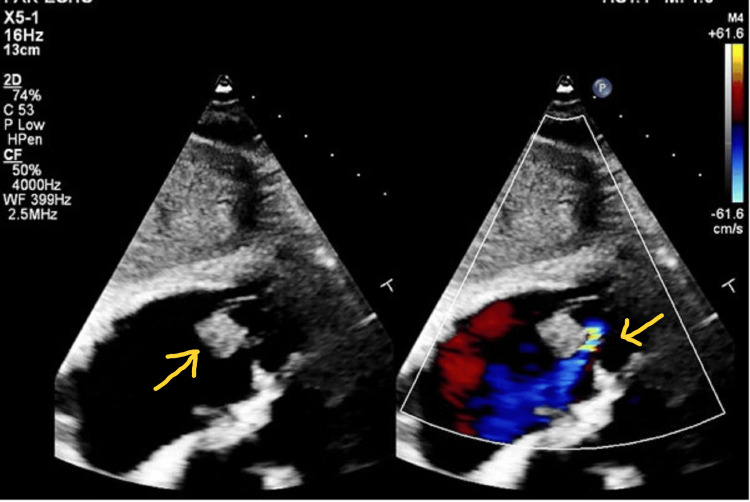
Transthoracic echocardiogram showing multiple tricuspid valve vegetations and tricuspid regurgitation (yellow arrows)
